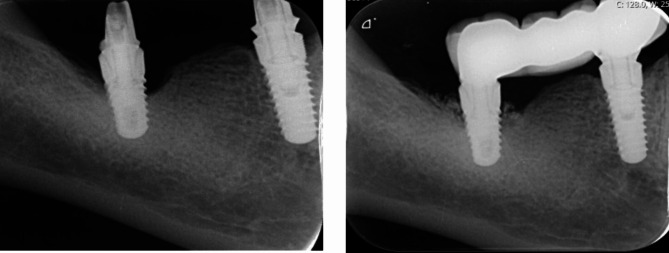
Radiographic view at baseline (left) and post-operative 12 months (right)
